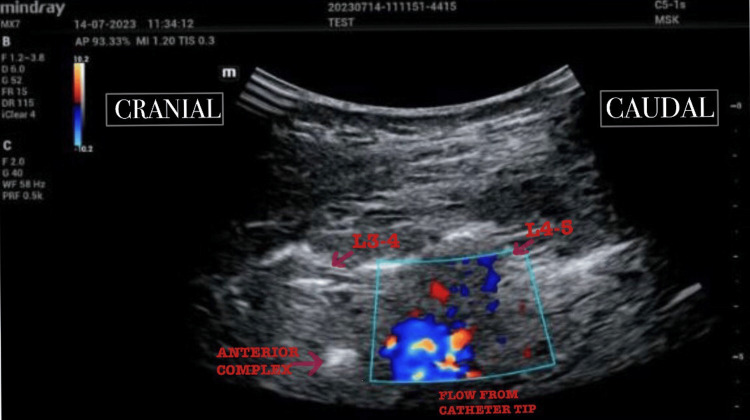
Flow from the catheter tip visualised in the L4 - L5 epidural space using colour flow Doppler
